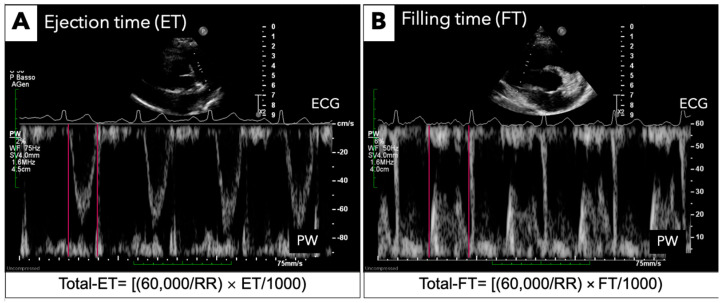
Total isovolumic time echocardiographic measurement. (A) RV total ejection time; (B) RV total filling time. Total isovolumic time is measured in seconds/minute.
